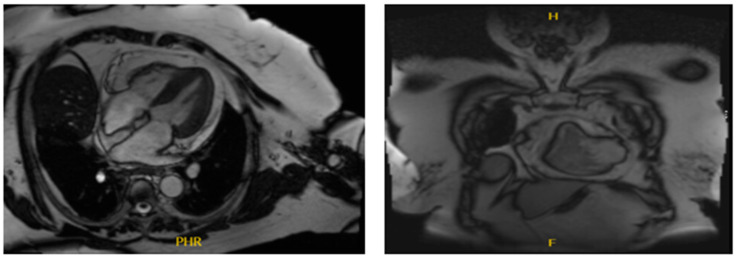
CV MRI showing a large intracardial mass (27 mm × 17 mm) in the mid-lateral LV wall with tissue characteristics suggestive of metastasis with normal biventricular size and systolic function.
